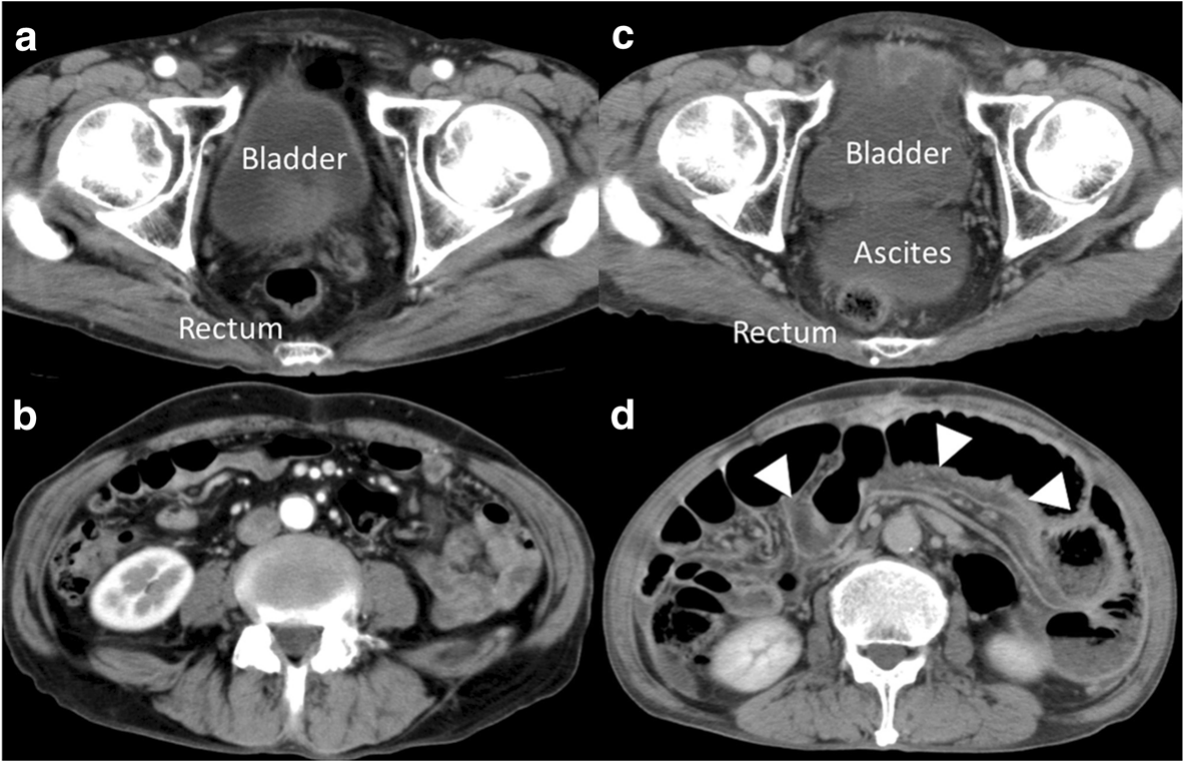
Computed tomography imaging showed the influence of peritoneal carcinomatosis. a The abdominal CT image before the abdominal surgery represents no findings of ascites. b The abdominal CT image before the abdominal surgery represents no findings of peritonitis. c Rectovesical excavation. d
